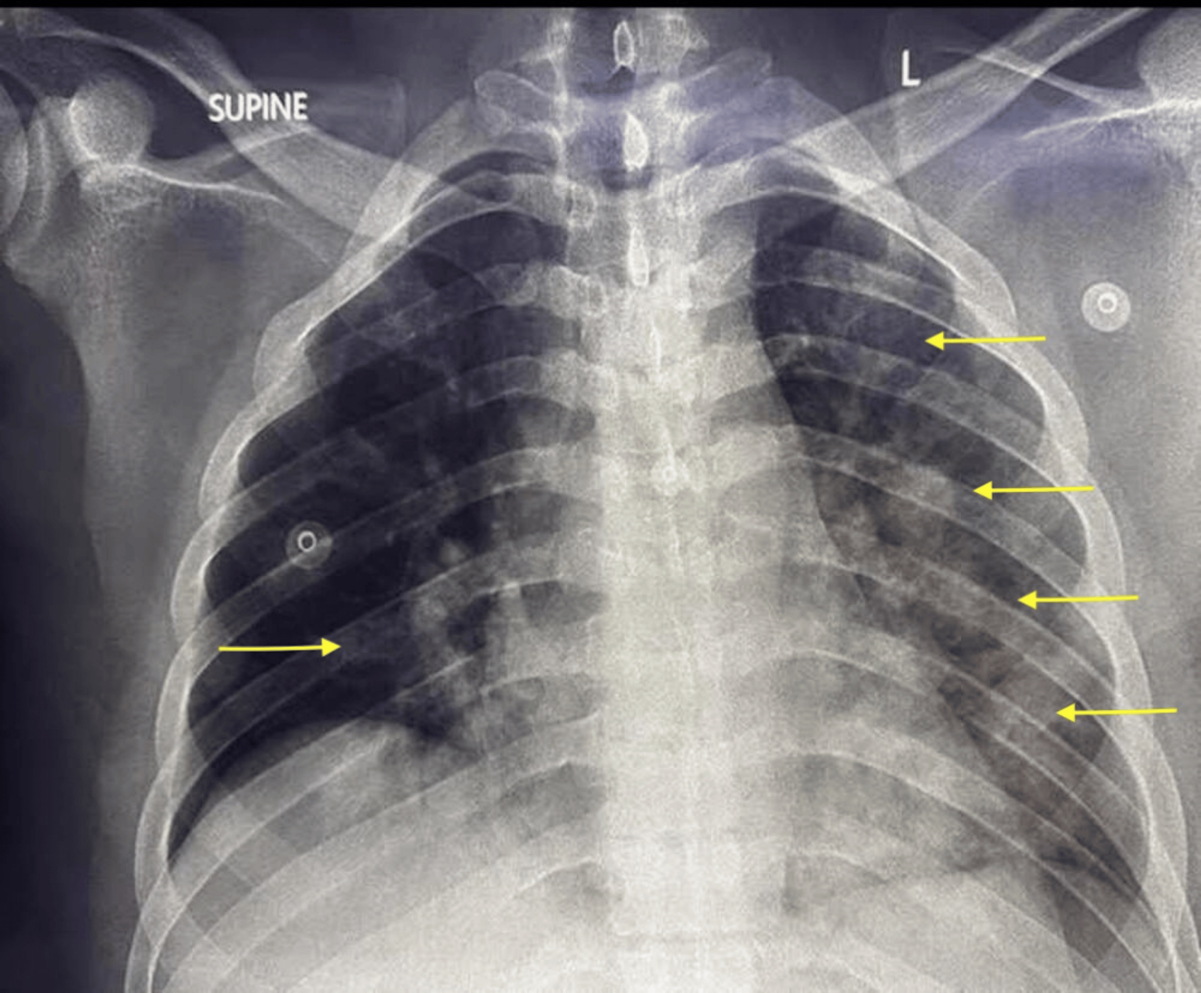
Follow-up chest radiograph indicating pulmonary oedema. The yellow areas indicate new hazy opacificities
